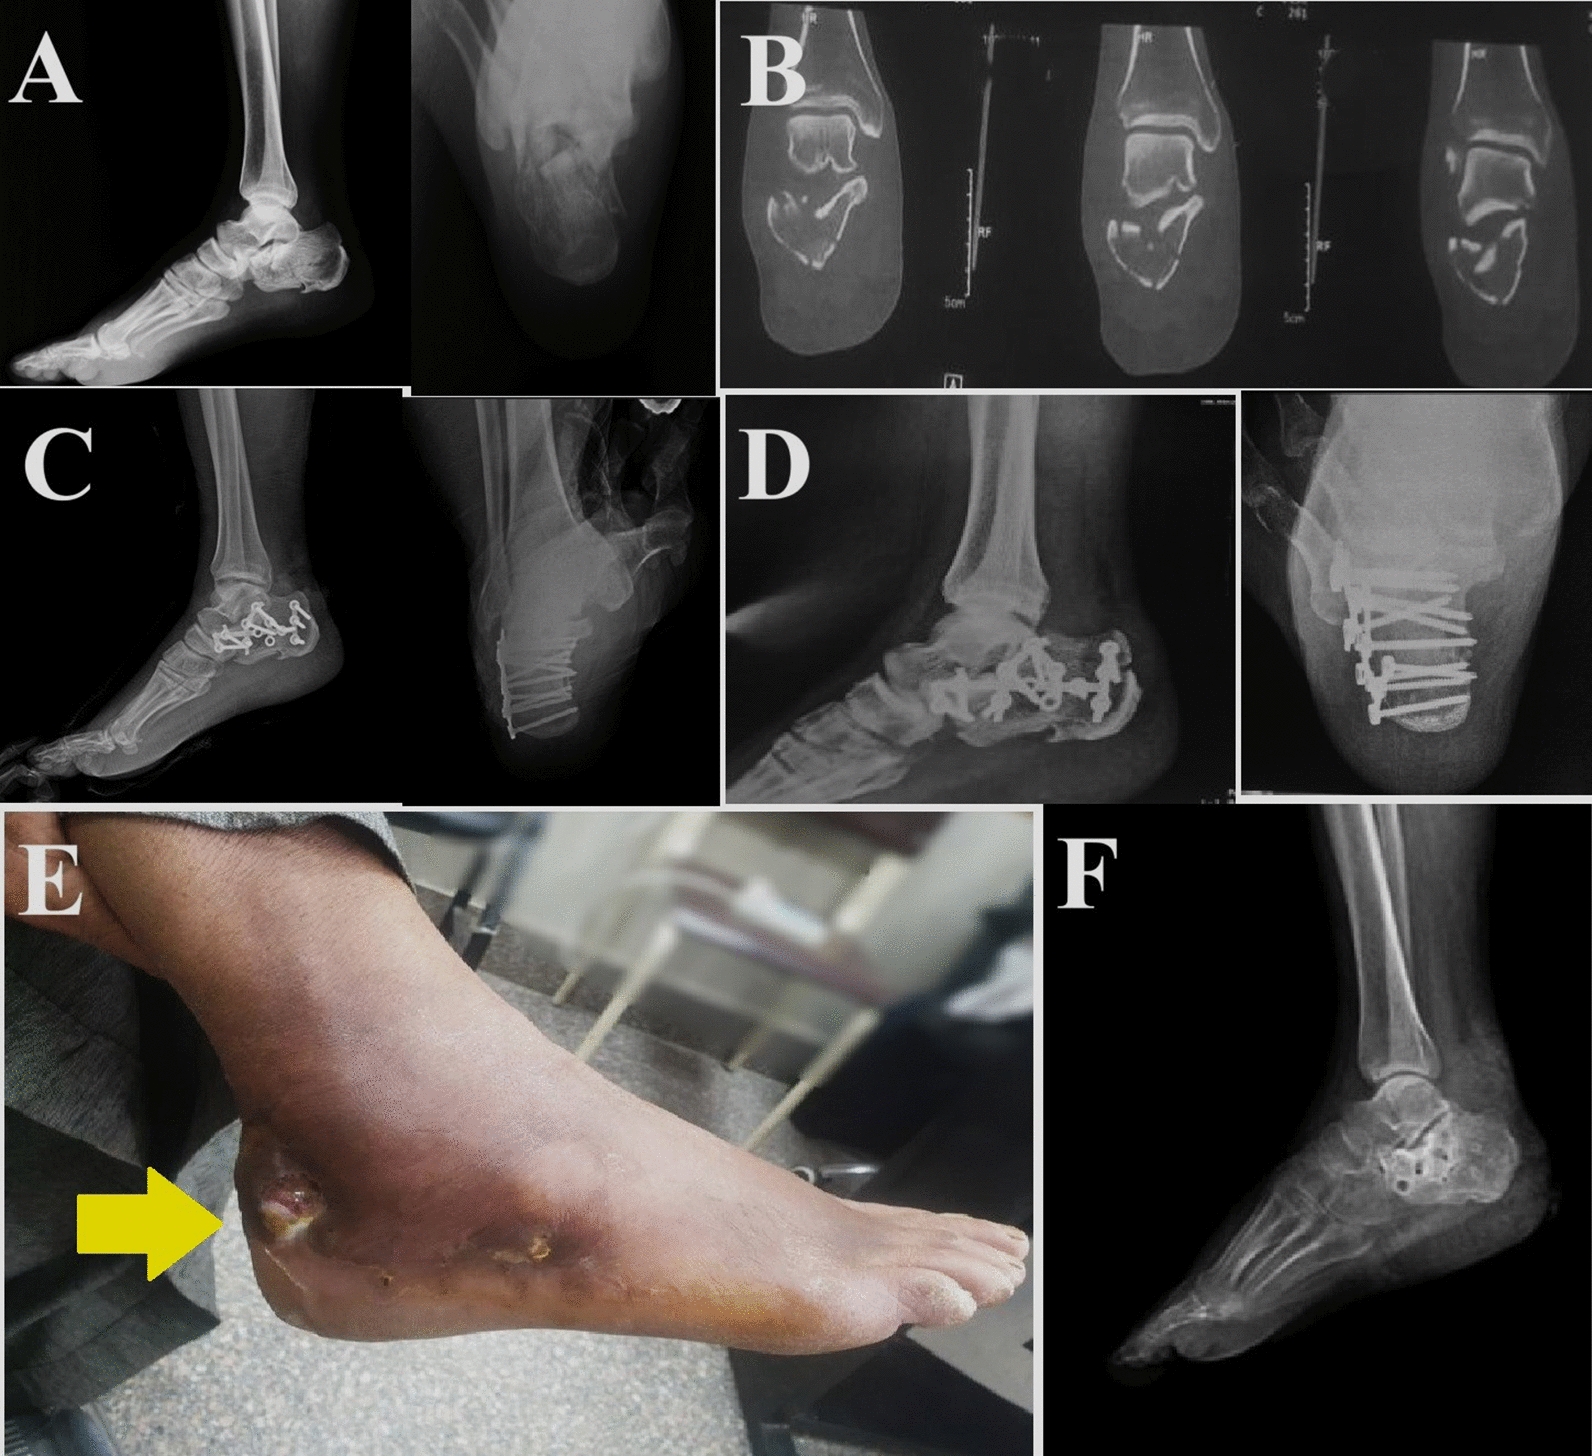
Male patient, 63 years old, presented with fractured left calcaneus, Sanders III, osteosynthesis by open reduction, and internal fixation through extensible lateral approach (ELA). A and B Preoperative images. C Immediate postoperative, and D Last follow up radiographs. E After 7 months of follow up, he presented with a sinus discharging pus (yellow arrowhead). F Debridement and metal removal were performed
